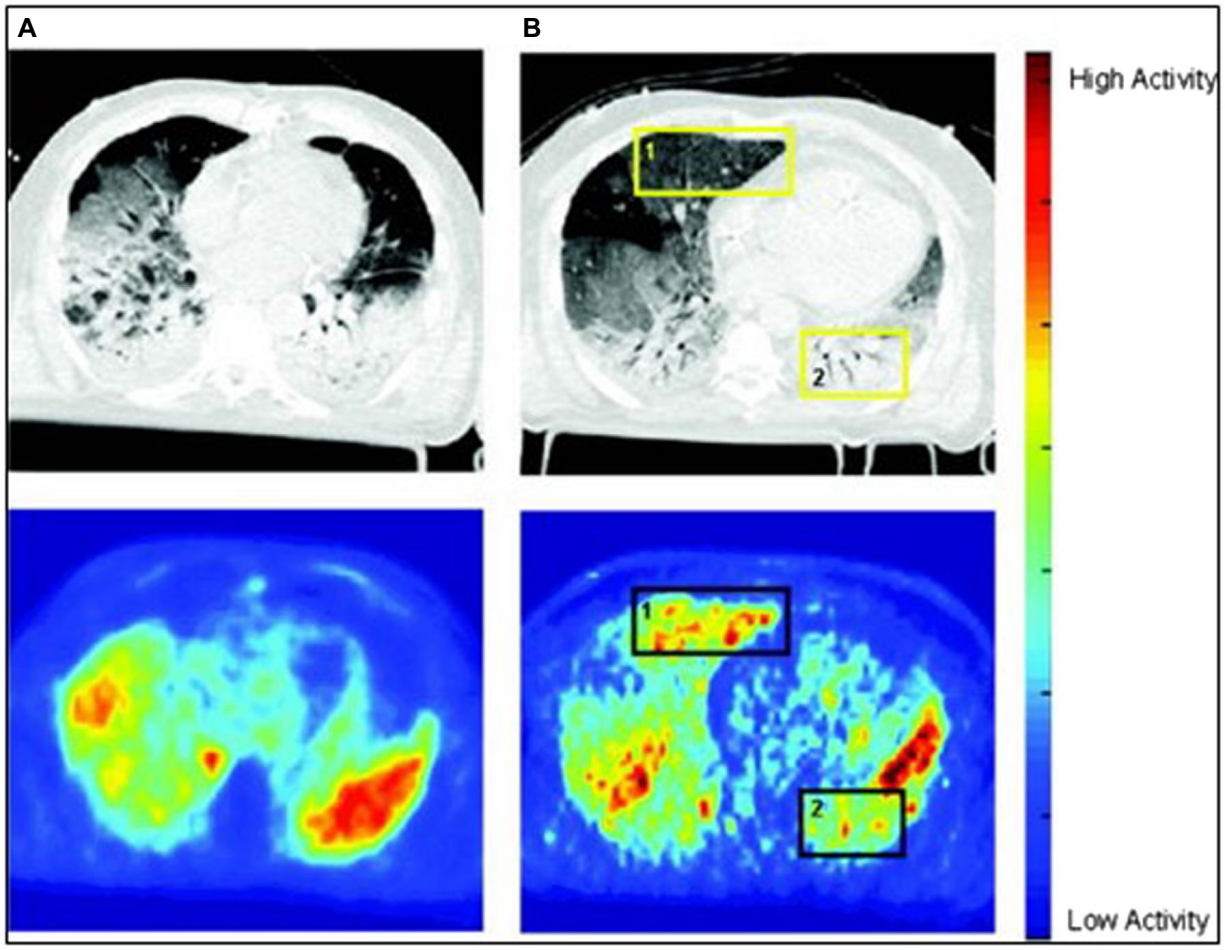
Computed tomography (CT) scans (upper row) and positron emission tomography (PET) scans of [^18^F]FDG uptake (bottom row) in two patients with acute respiratory distress syndrome (ARDS). In patient A, [^18^F]FDG uptake is highest in regions of increased lung density on CT, suggesting that these opacities are inflamed and possibly responsible for ARDS. In contrast, in patient B, there is a dissociation between [^18^F]FDG signal and density, with increased [^18^F]FDG uptake in nondependent normally aerated regions of both lungs. A hypothesis is that, in this case, the [^18^F]FDG signal mainly reflects ventilator-induced lung injury (VILI), as these are the regions exposed to mechanical ventilation (Reproduced from Bellani et al., 2009).
